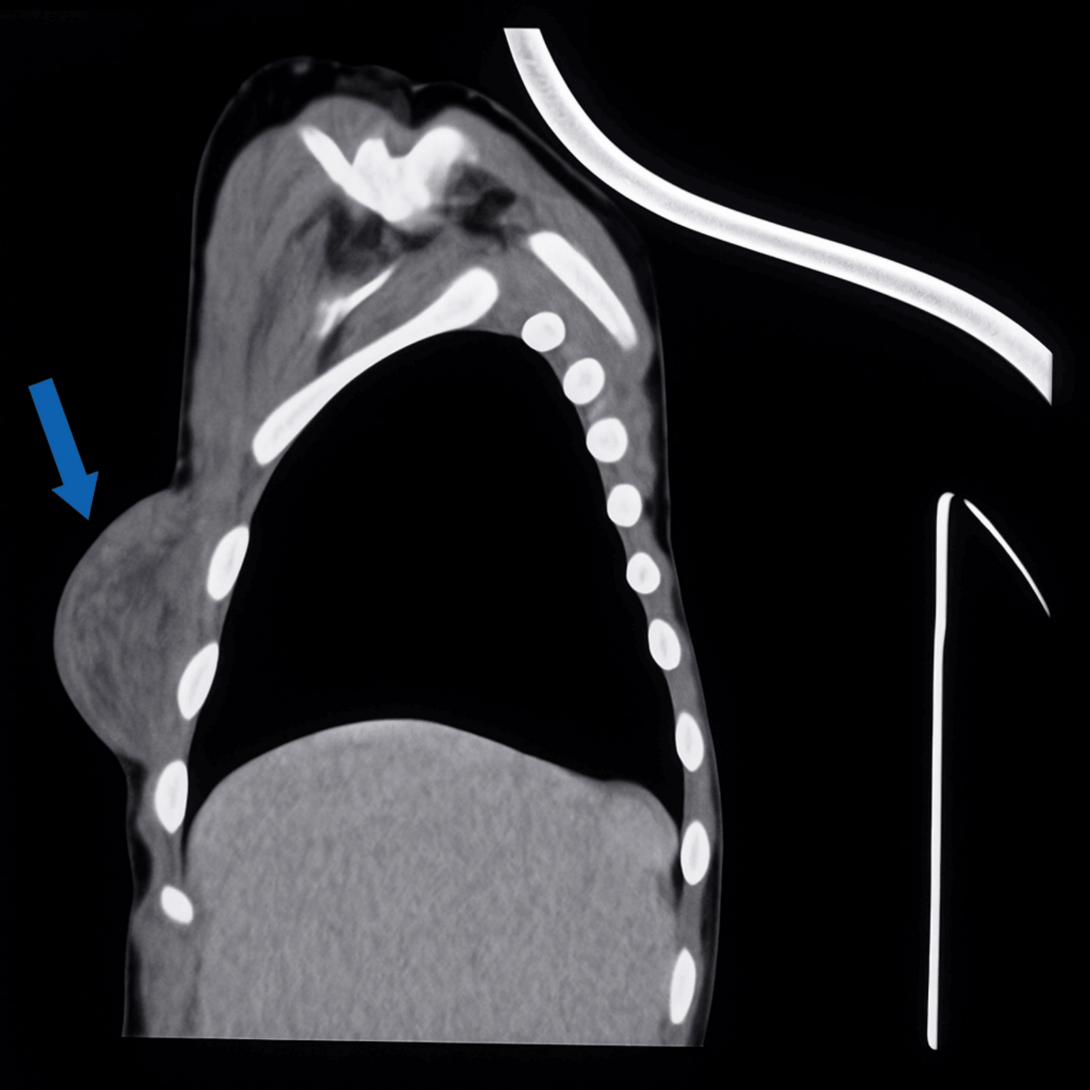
CT scan reveals a well-defined, encapsulated subcutaneous mass in the right chest wall.
